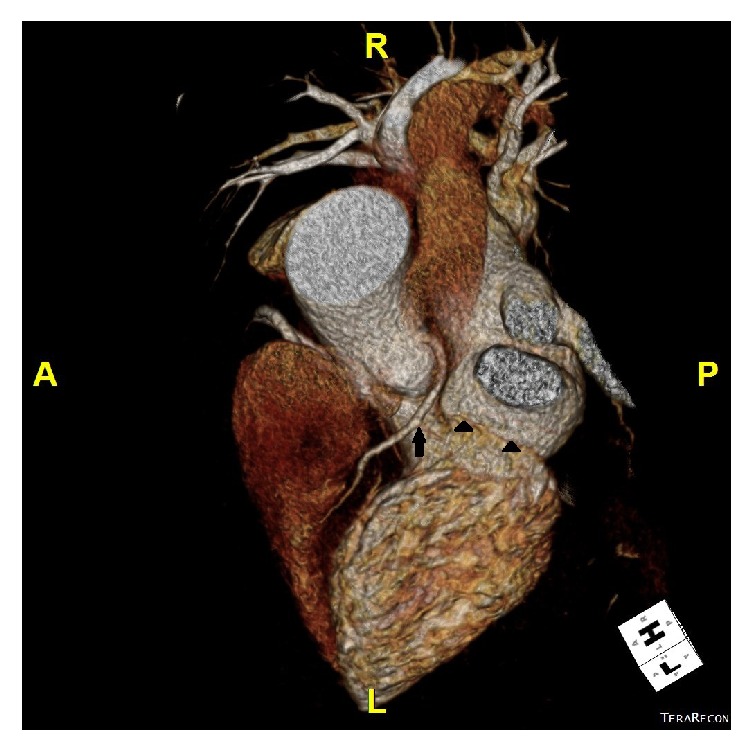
Top view three-dimensional computed tomography scan showing single left anterior descending artery (arrow) and absence of LCX in the atrioventricular groove (arrow heads).
